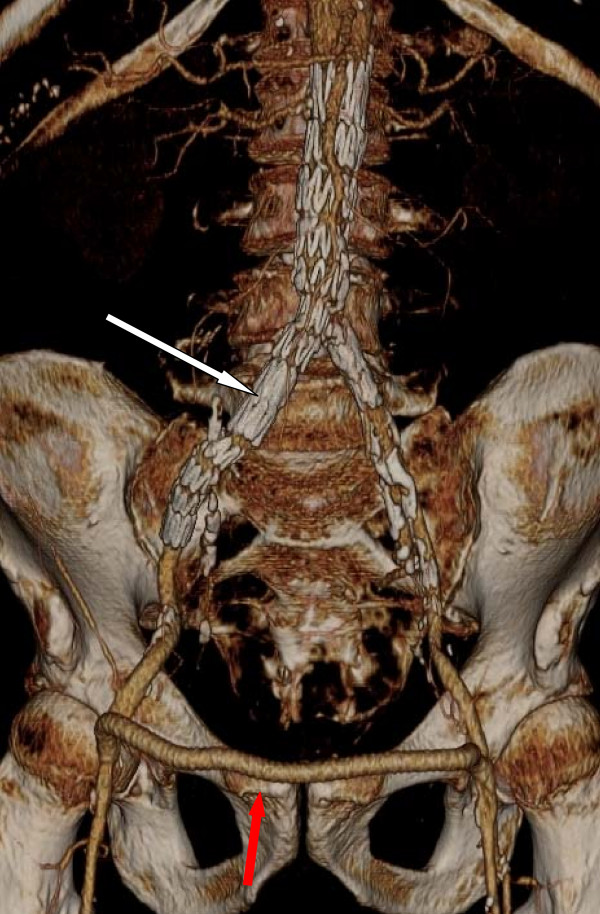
Postoperative CT. Postoperative CT 3-D reconstruction showing endovascular exclusion of the AV fistula with an aorto-uni-iliac graft (white arrow) and a functioning right to left femoro-femoral cross-over bypass (red arrow).
